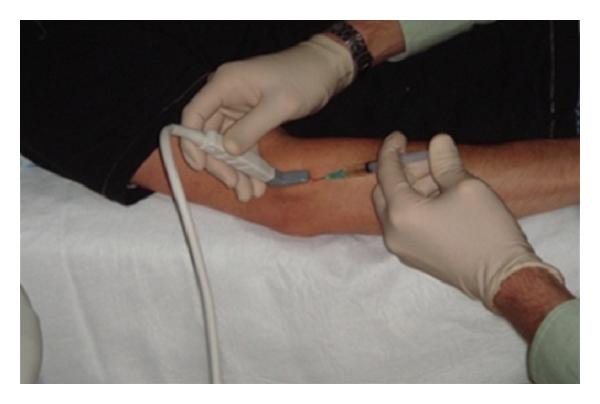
Injection of lateral epicondyle under ultrasound guidance.
